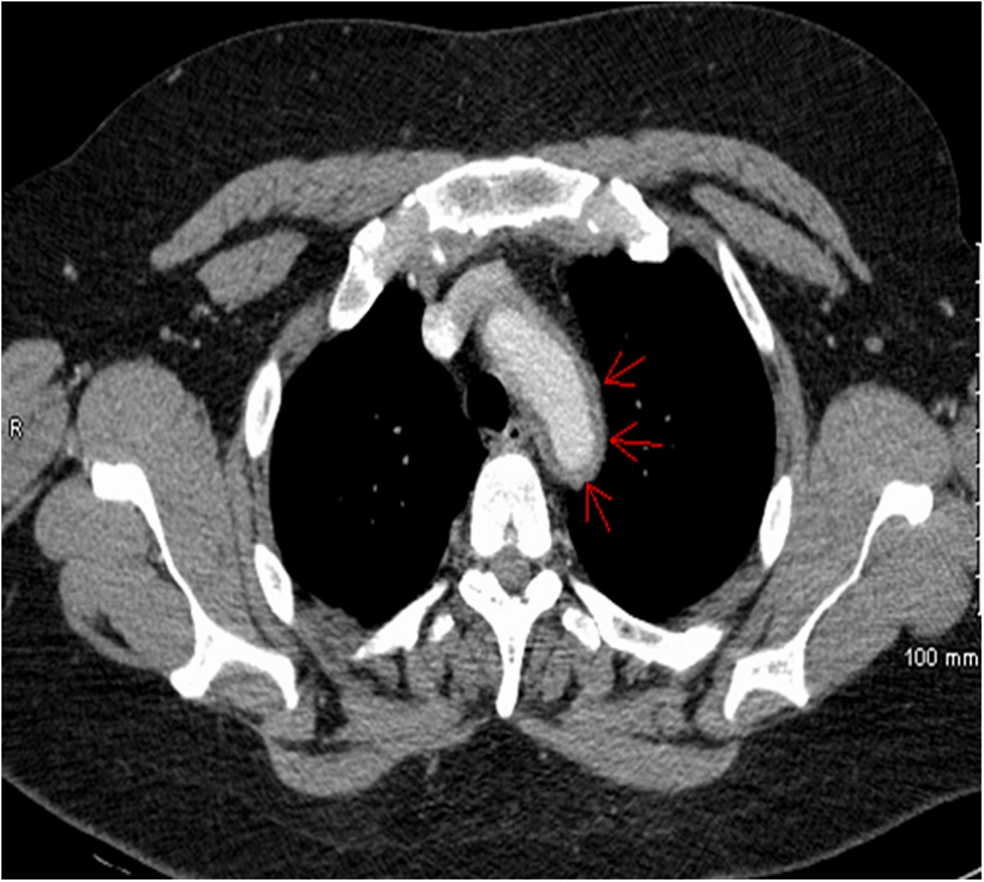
Computed tomography angiography of the chest, axial view The red arrow indicates mural thickening.
